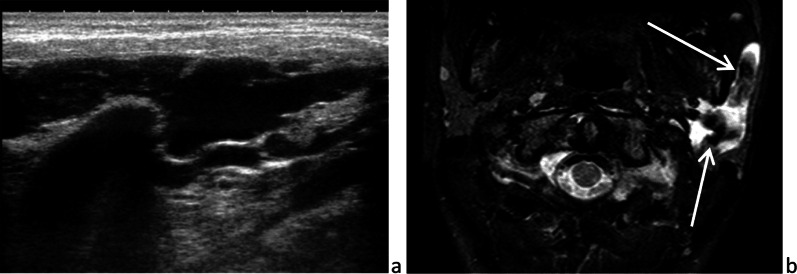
Venous malformation. Tortuous veins containing phleboliths, on sonography (a) and *T*_2_*-weighted image (b)
